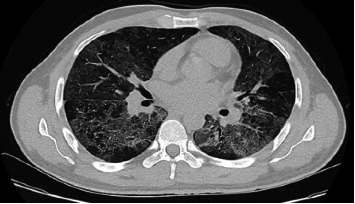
Bilateral ground glass opacities in both lungs from medial to peripheral areas on thoracic computed tomography scan.
